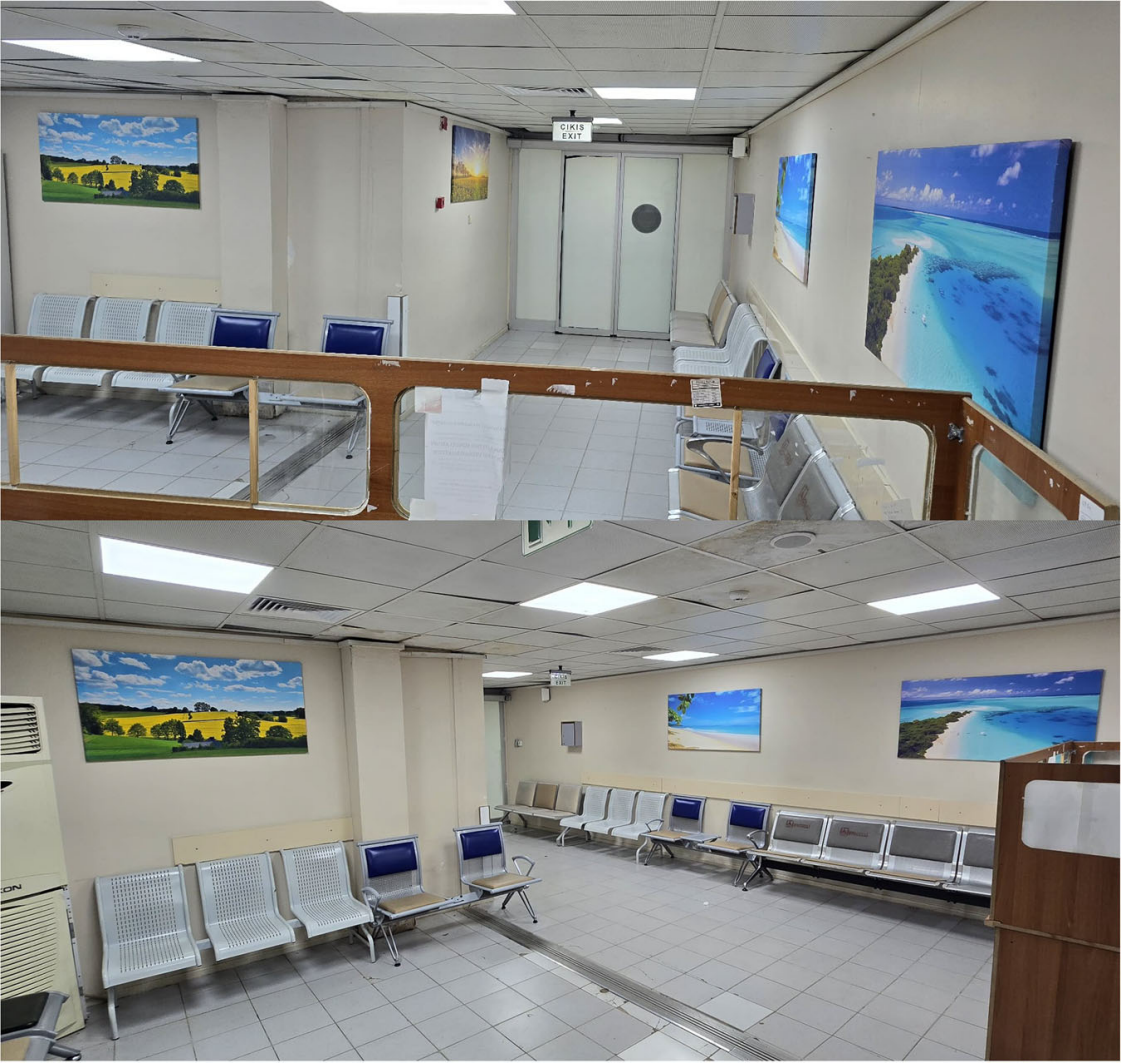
The appearance of the DWR, featuring calming visual elements and ambient music to create a relaxing environment for patients awaiting CCTA. CCTA, coronary computed tomography angiography
